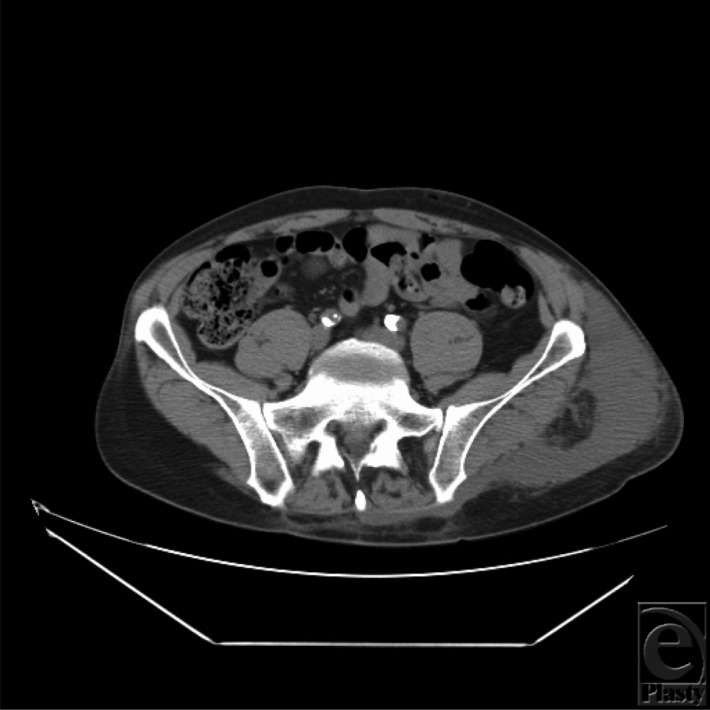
Magnetic resonance imaging axial image of the Morel-Lavallée lesion over the left trochanteric region with extension to the back.
